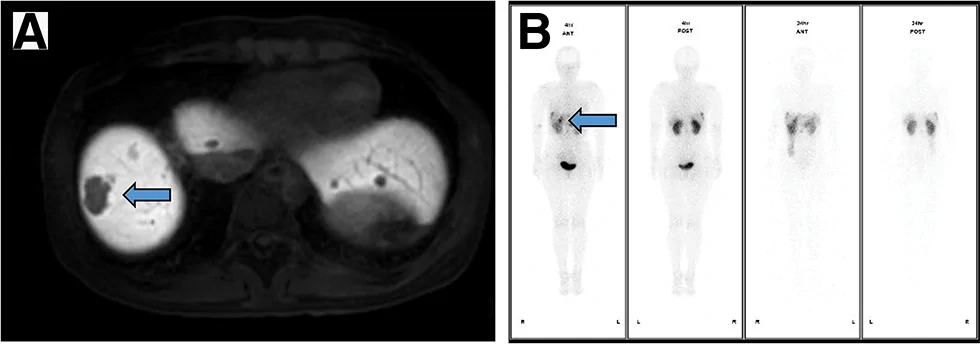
(A) Enlargement of hepatic metastases. (B) Corresponding abnormal uptake on octreotide scintigraphy.
